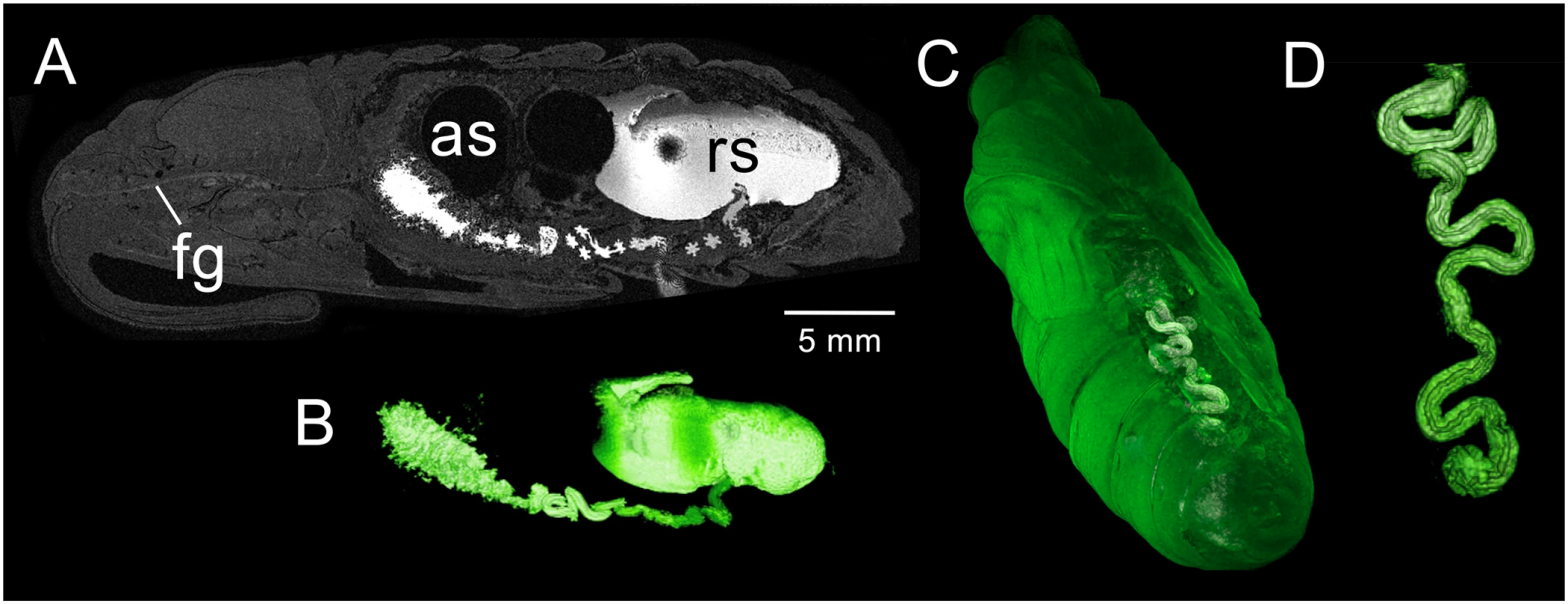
3D-GRE MR imaging data acquired from a *M*. *sexta* pupa 16–17 days after metamorphosis. (A) Sagittal section displaying the deflated midgut and enlarged rectal sac (rs). Abdominal air sacs (as) deform the anterior portion of the rectal sac. Midgut contents have been released into the hindgut. (B) 3D surface-rendering of the midgut, anterior hindgut and rectal sac. (C) Ventral 3D surface-rendering showing the position of the anterior hindgut. (D) 3D surface-rendering of the coiled anterior hindgut.
